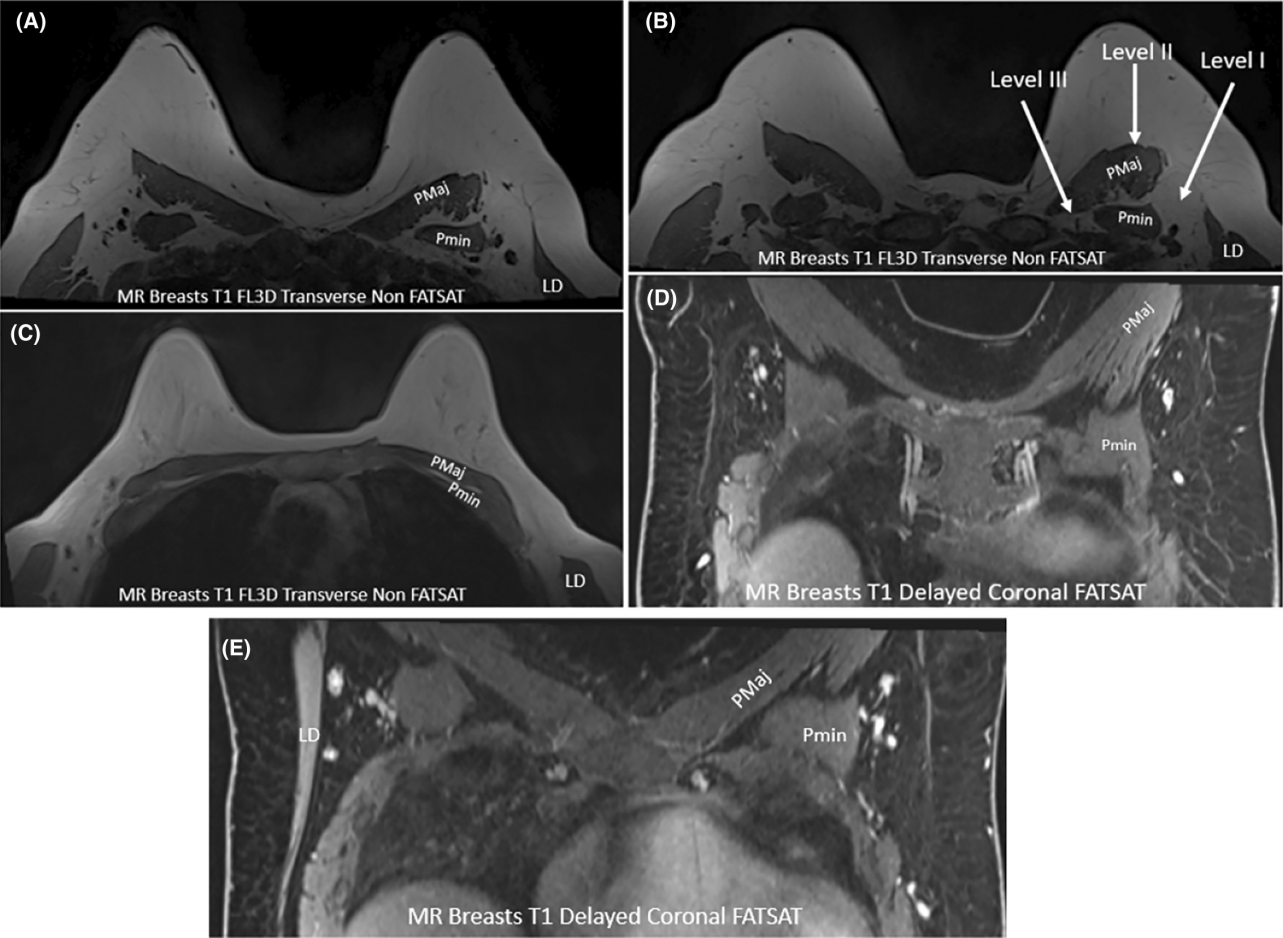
Magnetic resonance (MR) imaging of the breast and axilla. (A–C) Transverse MR images of different breasts, demonstrating variation in the appearances of the pectoral and latissimus dorsi muscles, which can be used as landmarks to identify the three axillary levels. (D, E) Coronal MR images of the chest wall and axilla region to allow identification of the levels of the axilla. (D) is acquired more anteriorly, and (E) is acquired slightly more posteriorly as the latissimus dorsi muscle observed laterally. LD, latissimus dorsi muscle; PMaj, pectoralis major muscle; Pmin, pectoralis minor muscle.
